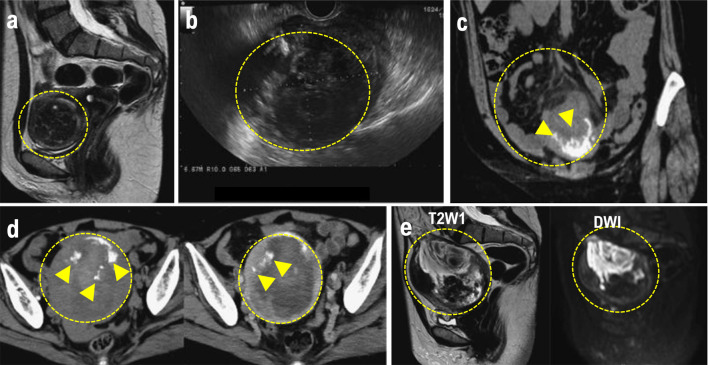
(a) MRI performed at the initial consultation. The mass exhibited a clearly demarcated low signal intensity on T2W1 and was diagnosed as a uterine fibroid. (b) Transvaginal ultrasound after lower gastrointestinal endoscopy revealed a 90 × 69 mm mass in the uterine body, with a heterogeneous interior and irregular margins. (c) Plain CT. Increased density was observed in the mesenteric fat tissue surrounding the mass. A mass with marginal calcification was found on the anterior wall of the uterine body, protruding cephalad. (d) Comparison of the initial CT (left) with a CT performed 8 days later for preoperative evaluation (right). The uterine mass had shrunk from 10 × 11 cm (left panel) to 10 × 9.5 cm (right panel), and the absorption value of the internal high-density area had decreased, suggesting absorption of the hematoma. (e) MRI for further investigation of suspected uterine sarcoma. The mass showed moderate signal intensity on T2W1 and high signal intensity on DWI, and heterogeneous signals were present within it, suggesting hemorrhage and necrosis. The mass is indicated by the yellow dotted circle. Heterologous components including osteosarcoma and chondrosarcoma are indicated by the white arrowheads. CT: computed tomography; MRI: magnetic resonance imaging.
